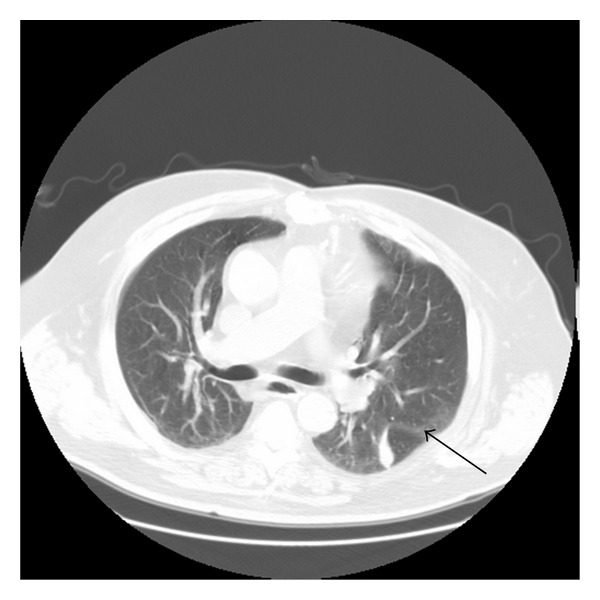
Chest computed tomogram with intravenous contrast two months prior to admission revealing atelectasis of the left lower lobe (arrow).
